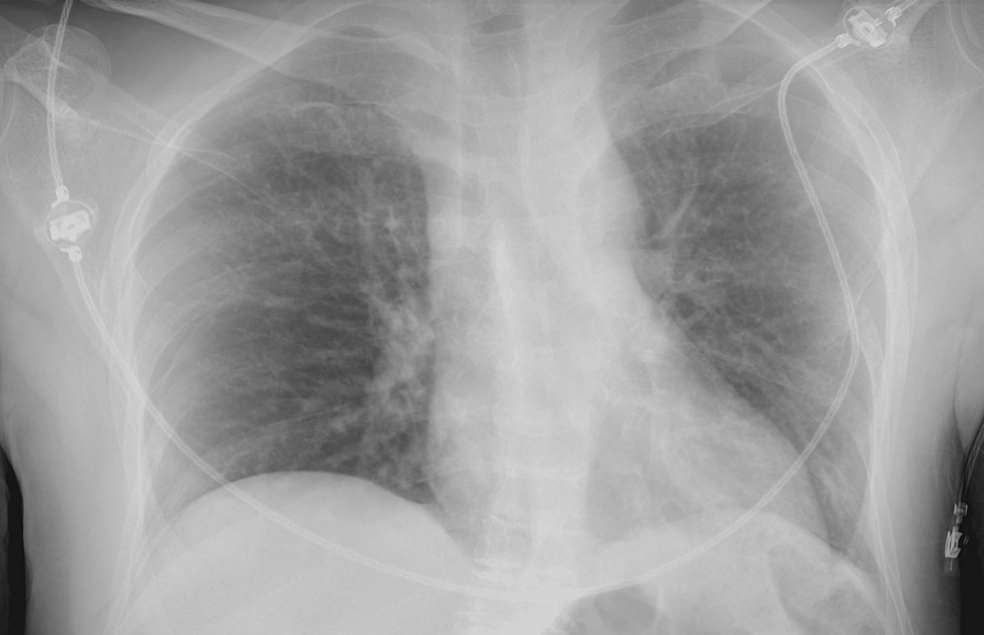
Chest radiograph - anteroposterior view.
